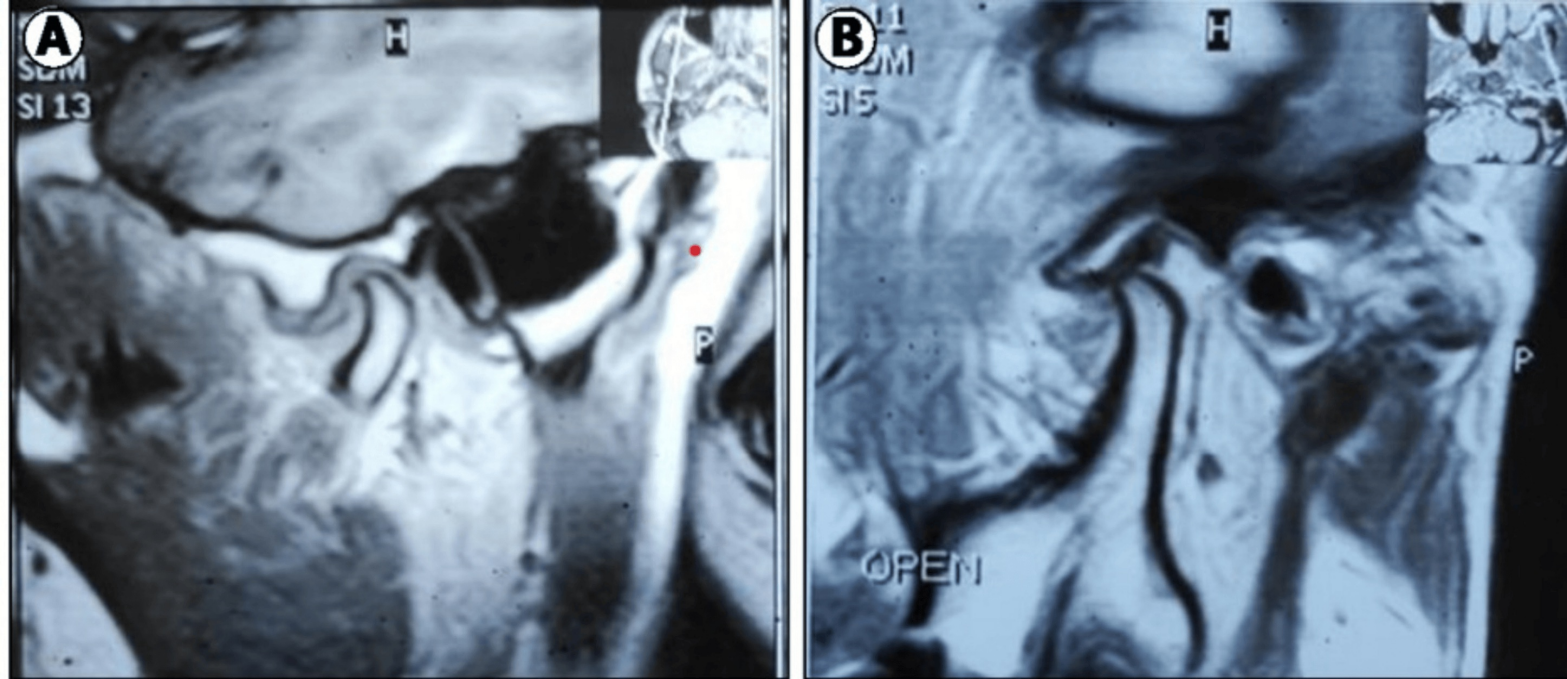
Preoperative MRI images. A: Closed mouth; B: open mouth
